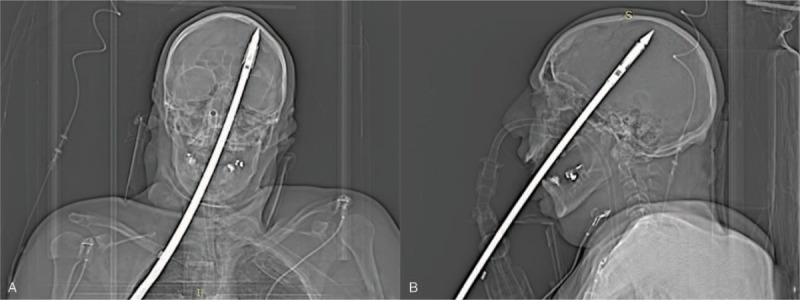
3D CT image reconstruction. The metallic object penetrated the oral cavity and crossed the left hard palate, nasal cavity, pterygoid process, and the sphenoid hemisphere, orbital apex region, orbital fissure, and the left optical channel.
